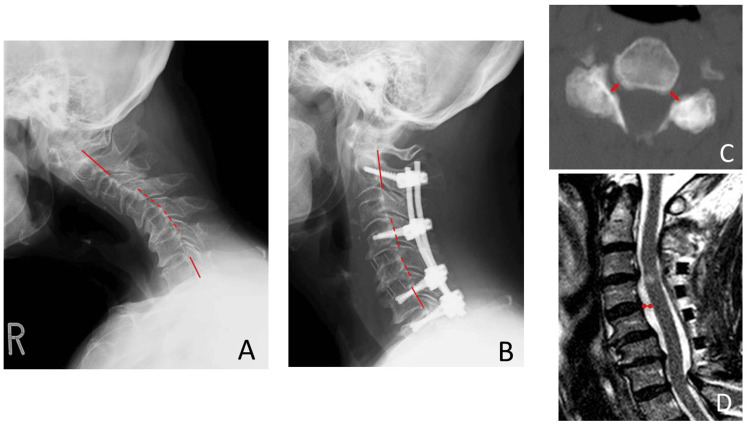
Radiological assessments. (A) Preoperative C2–7 angle (solid line) and C4–5 local angle (dashed line). (B) Postoperative C2–7 angle (solid line) and C4–5 local angle (dashed line). (C) Anteroposterior diameter of the C4–5 intervertebral foramen. (D) Posterior shift of the spinal cord on MRI.
